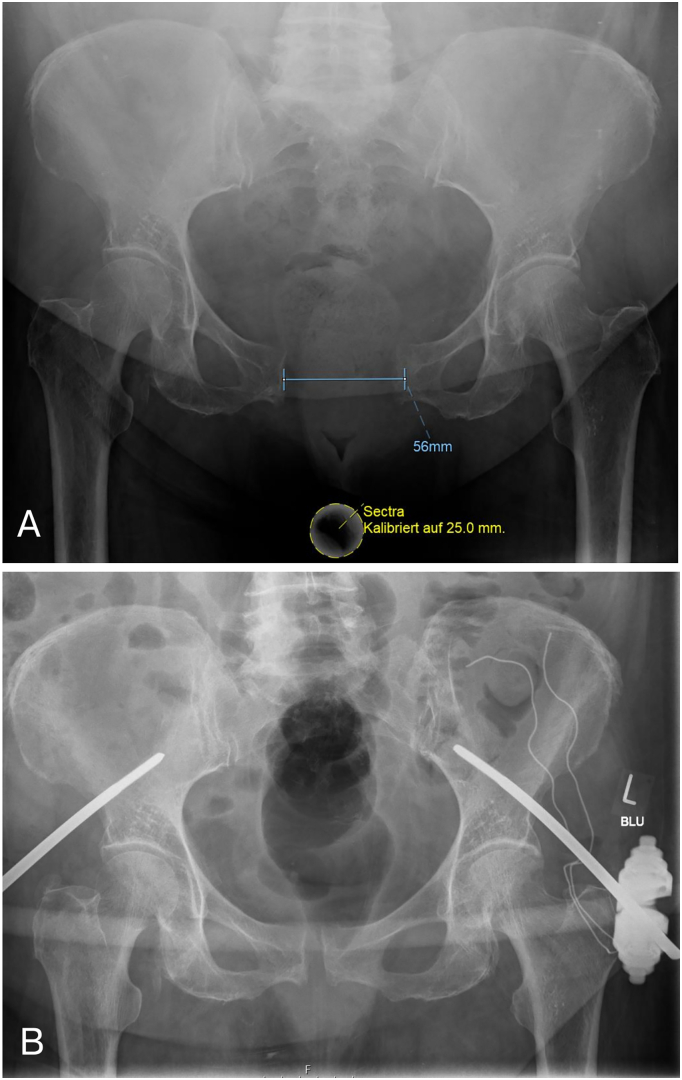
A: Shows the posttraumatic X-ray, a.p. of the pelvis with a diastasis of 56 mm. B: Shows the postoperative X-ray, a.p. after emergency stabilization using an external fixator of the pelvis.
